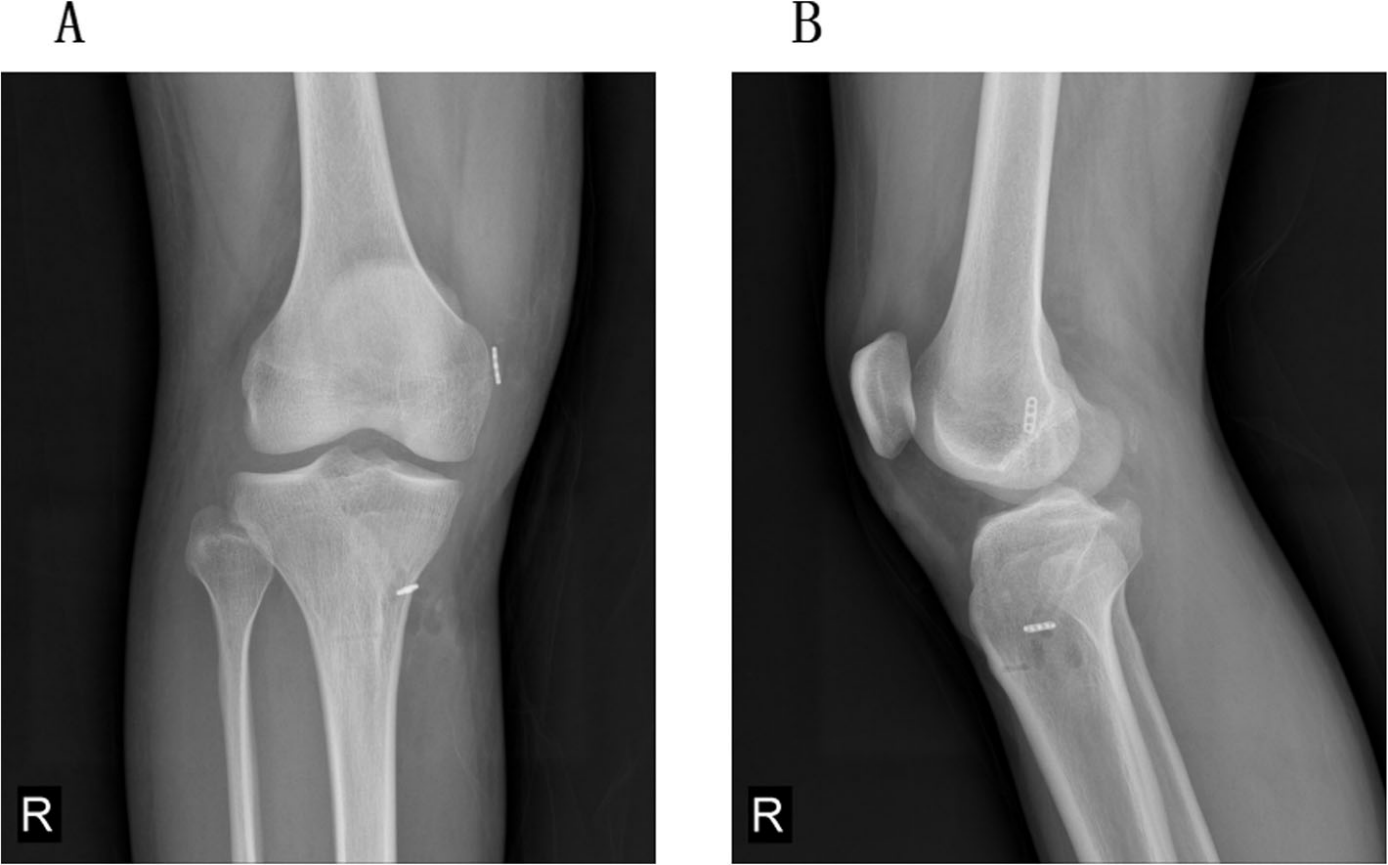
The postoperative X-ray showed an endobutton plate (Smith & Nephew) outside the tibial bone tunnel, which was used to fix the suture line passed through PRMM area. a anteroposterior film. b lateral film.  PRMM, posterior root of medial meniscus
